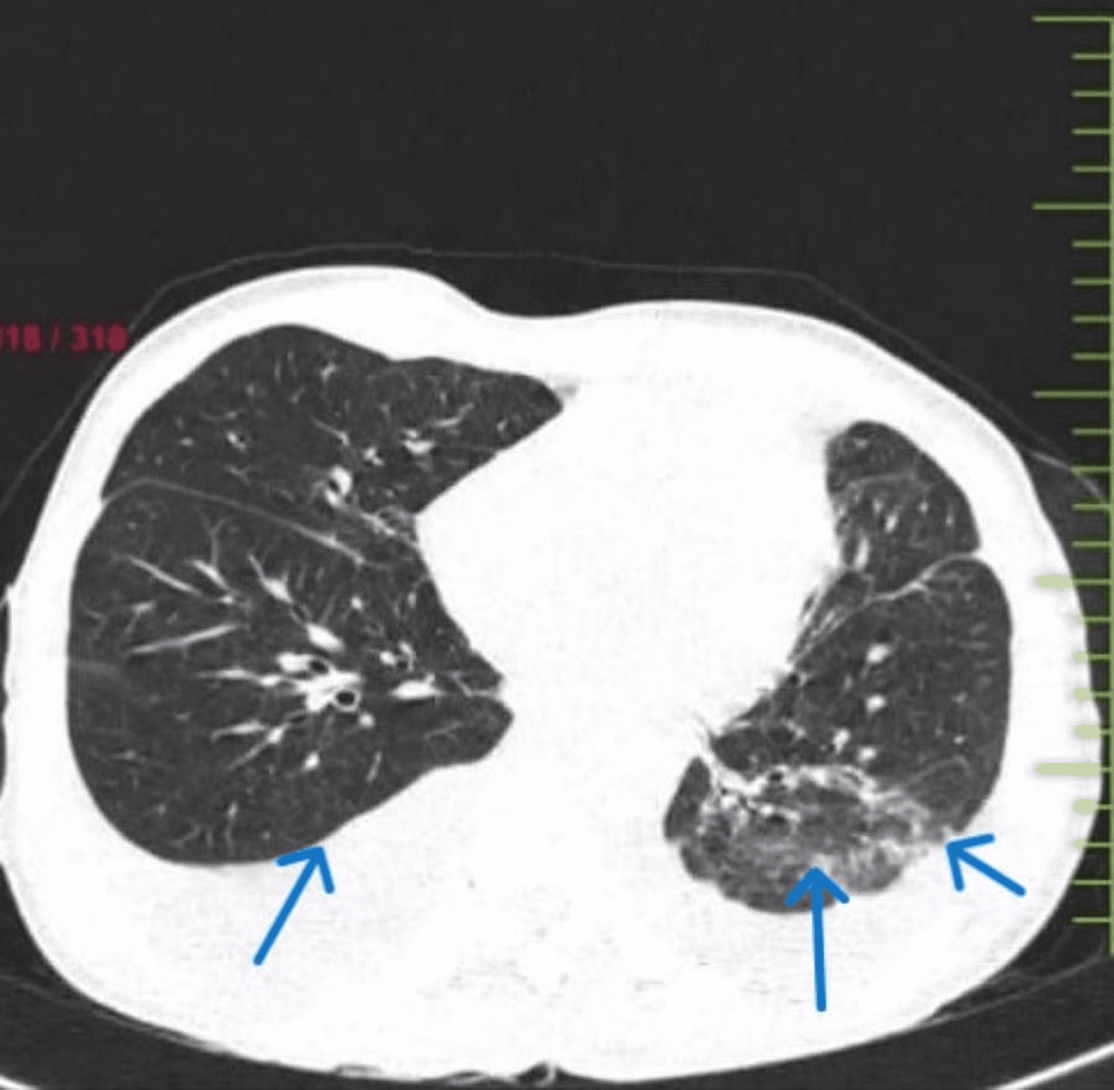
High-resolution computed tomograph (HRCT) of the thorax showing subcutaneous emphysema on the right side with bilateral pleural effusion with sub-segmental atelectasis and patchy areas of consolidation in lateral and posterior segments of the left lower lobe.
